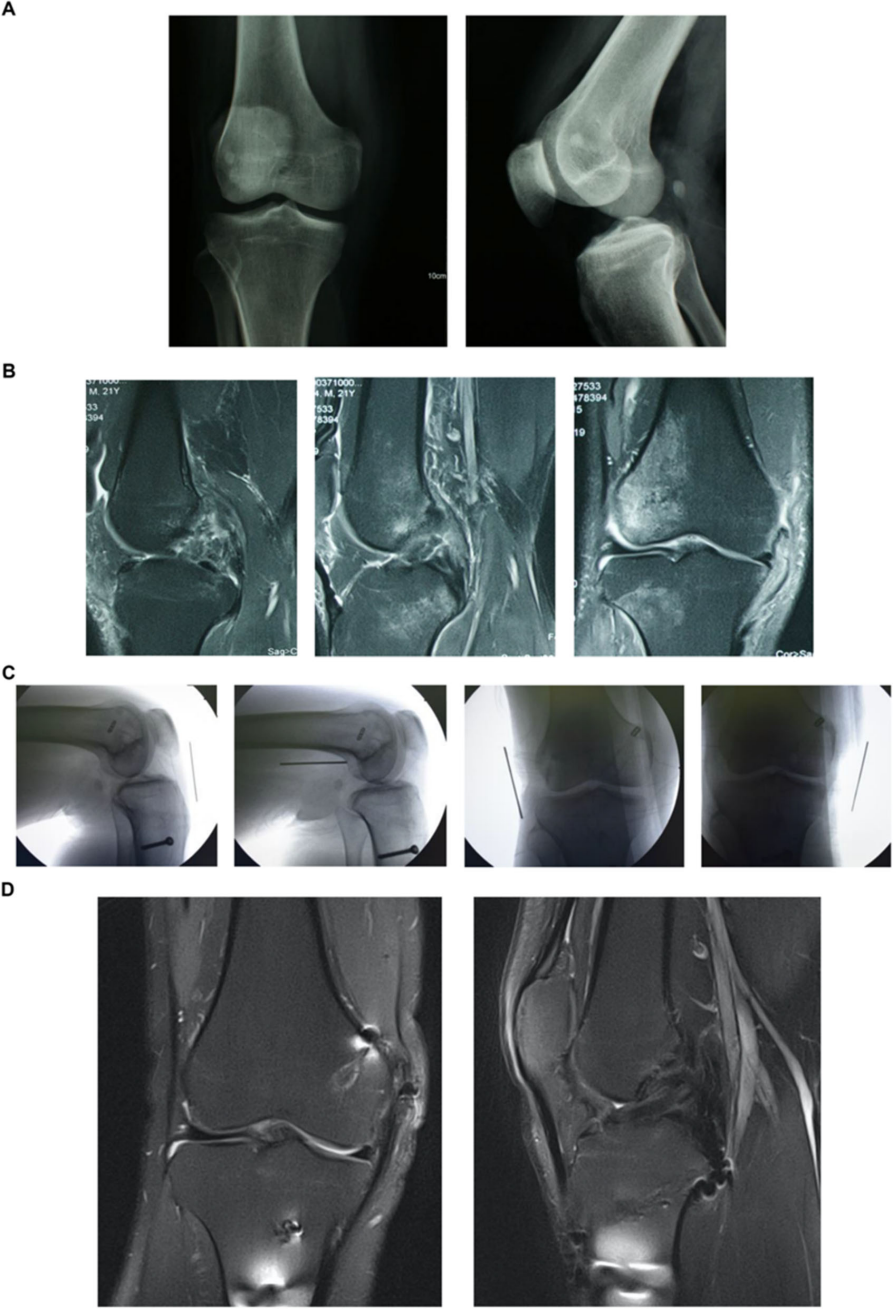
(A-D): A 21-year-old male patient suffered painful swelling of the right knee with functional obstruction for more than 2 months. Clinical diagnosis was chronic stage complicated right knee dislocation (KD-III: ACL + PCL + MCL) with bone contusion, no fracture or cartilage injury, and accompanied by medial and lateral meniscus injury (A, B). The surgery performed included arthroscopic probing in the right knee + ACL/PCL reconstruction + MCL repairing + medial and lateral meniscus repair. An X-ray stress examination showed that the knee maintained good stability at the last follow-up. The appearance of the ligament repair (ACL, PCL) and reconstructions (MCL) were as expected at 1 year after surgery (C, D). Functional scores after 1 year were International Knee Documentation Committee score: 73; Lysholm score: 85; and Tegner score: 5
